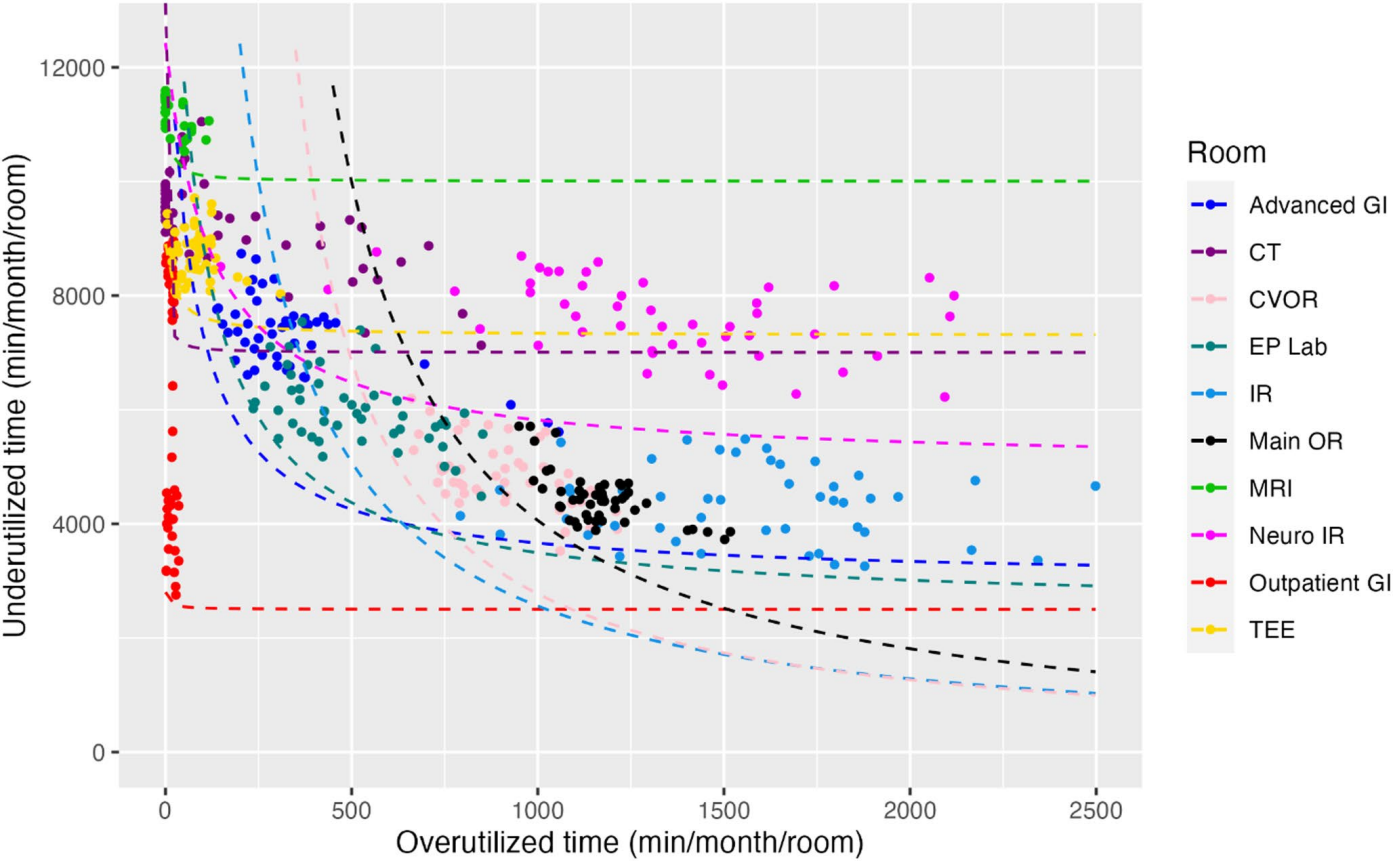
Performance frontiers added for each location plotted in Fig. 1. CT, computed tomography; CVOR, cardiovascular operating rooms; EP, electrophysiology; GI, gastroenterology; IR, interventional radiology; MRI, magnetic resonance imaging; OR, main operating rooms; TEE, transesophageal echocardiography
